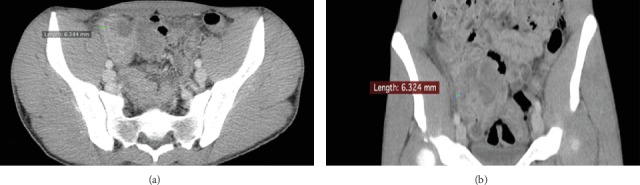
CT of the abdomen showing a thick-walled caecum, with adjacent fat stranding and a small volume of adjacent free fluid: (a) axial section and (b) coronal section.
